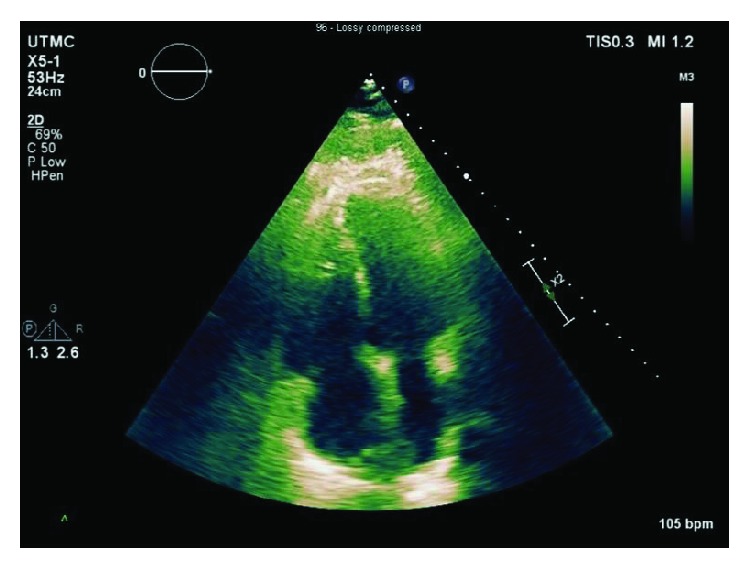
Transthoracic echocardiography one week later showing improvement in right ventricular size and function.
